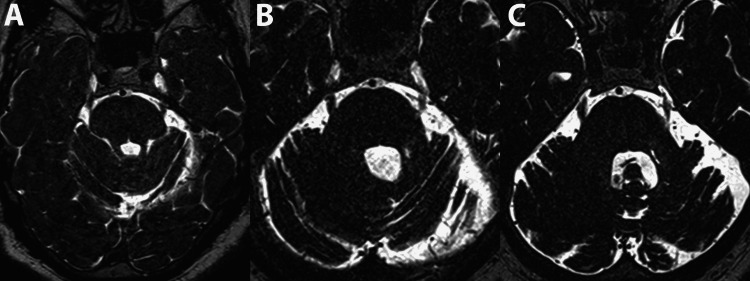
Radiographic progression. Axial FIESTA magnetic resonance imaging series from (A) initial diagnosis of trigeminal neuralgia, (B) following GKRS (Stockholm, Sweden: Elekta AB) treatment, and (C) following ZAP-X (San Carlos, CA: ZAP Surgical Systems, Inc.) GRS treatment. Atrophy within the distal left retrogasserian trigeminal nerve is noted within the post-GRS imaging. FIESTA: fast imaging employing steady-state acquisition; GKRS: Gamma Knife radiosurgery; GRS: gyroscopic radiosurgery
